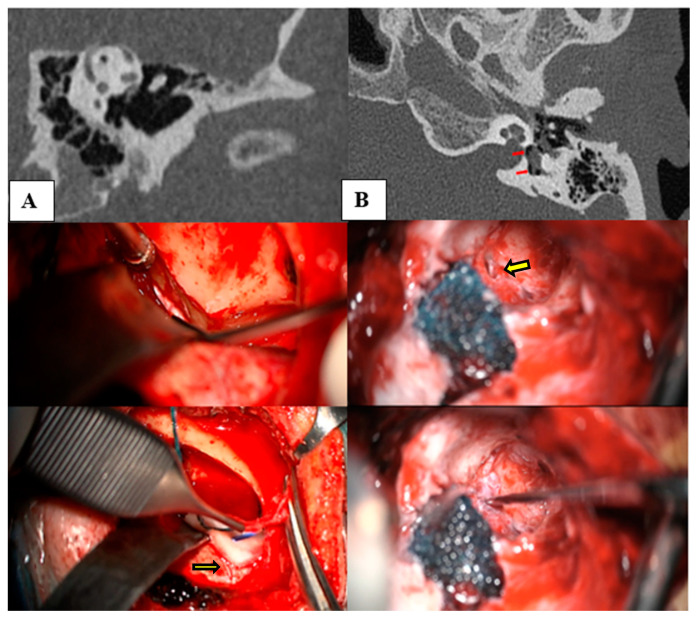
(A) (Left column) depicts the sequence of a middle fossa approach to cover the defect generated by a superior semicircular canal dehiscence (as seen in the CT scan). This approach includes the identification of the arcuate eminence, localization of the dehiscent canal (yellow arrow), and subsequent obliteration with autologous fascia. (B) (right column) depicts a retroauricular approach for sealing a perilymphatic fistula, which can be observed (red arrows in CT scan and yellow arrow intraoperative situation) with its levels of pneumolabyrinth in the vestibule, followed by its subsequent correction. Initially, autologous fascia was used, followed by reinforcement with autologous fat and Tissuecol.
